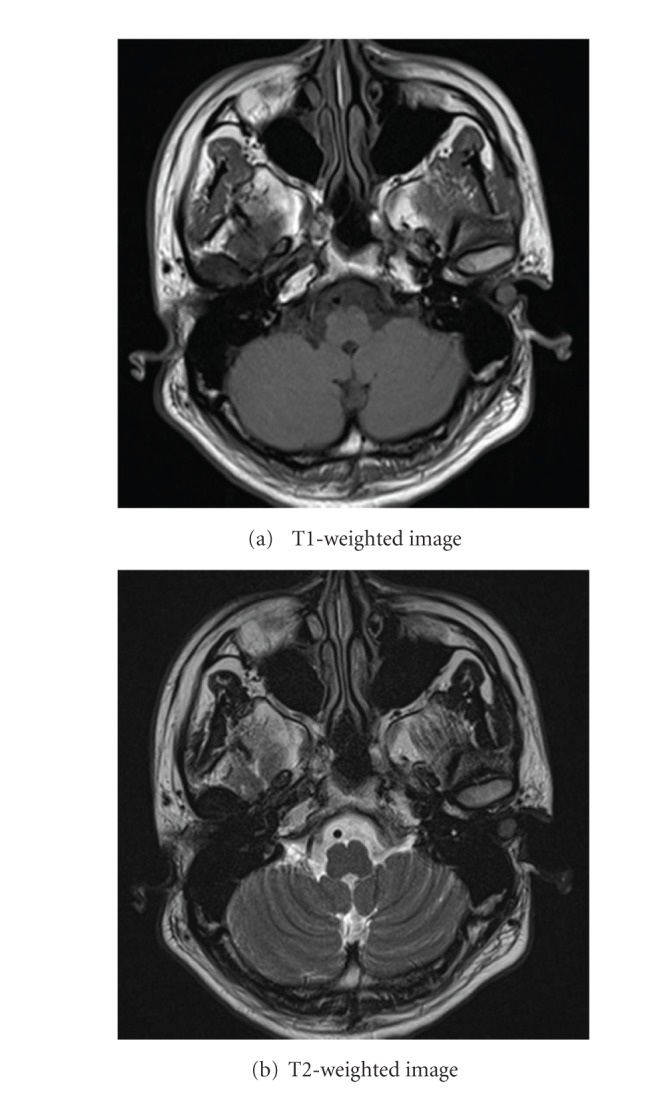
The MR findings: the mass measuring 10X10 mm showed a homogenous, iso signal intensity on a both T1- and T2-weighted image.
